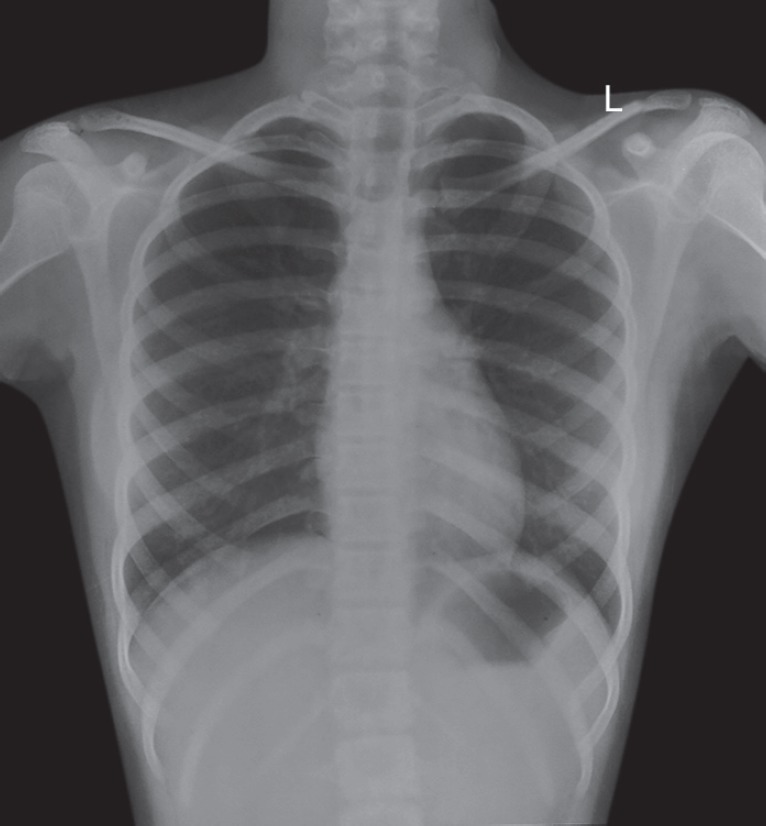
X-ray of the chest showing an enlarged heart, suggestive of cardiac involvement
